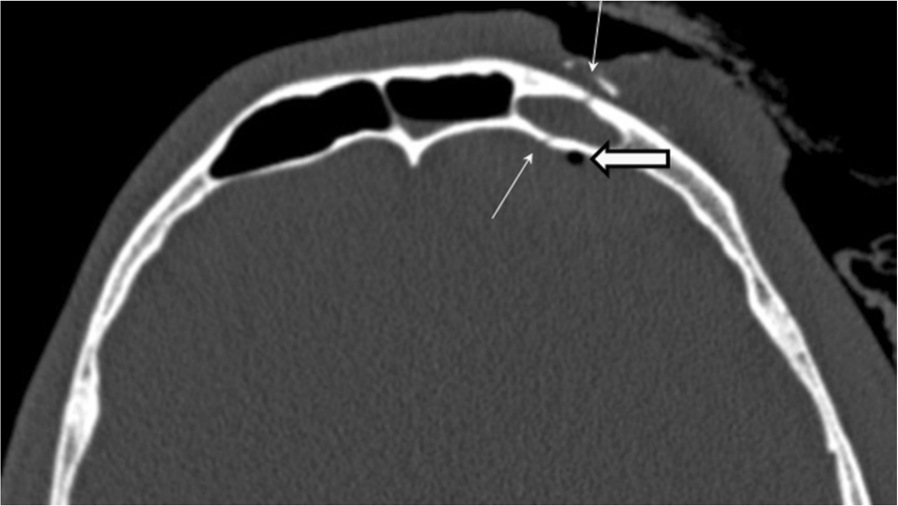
Frontal sinus fracture. Axial unenhanced CT image of the frontal bone demonstrates a nondisplaced fracture of the anterior and posterior walls (thin arrows) of the left frontal sinus, with partial opacification of the frontal sinus and small foci of pneumocephalus (thick arrow)
